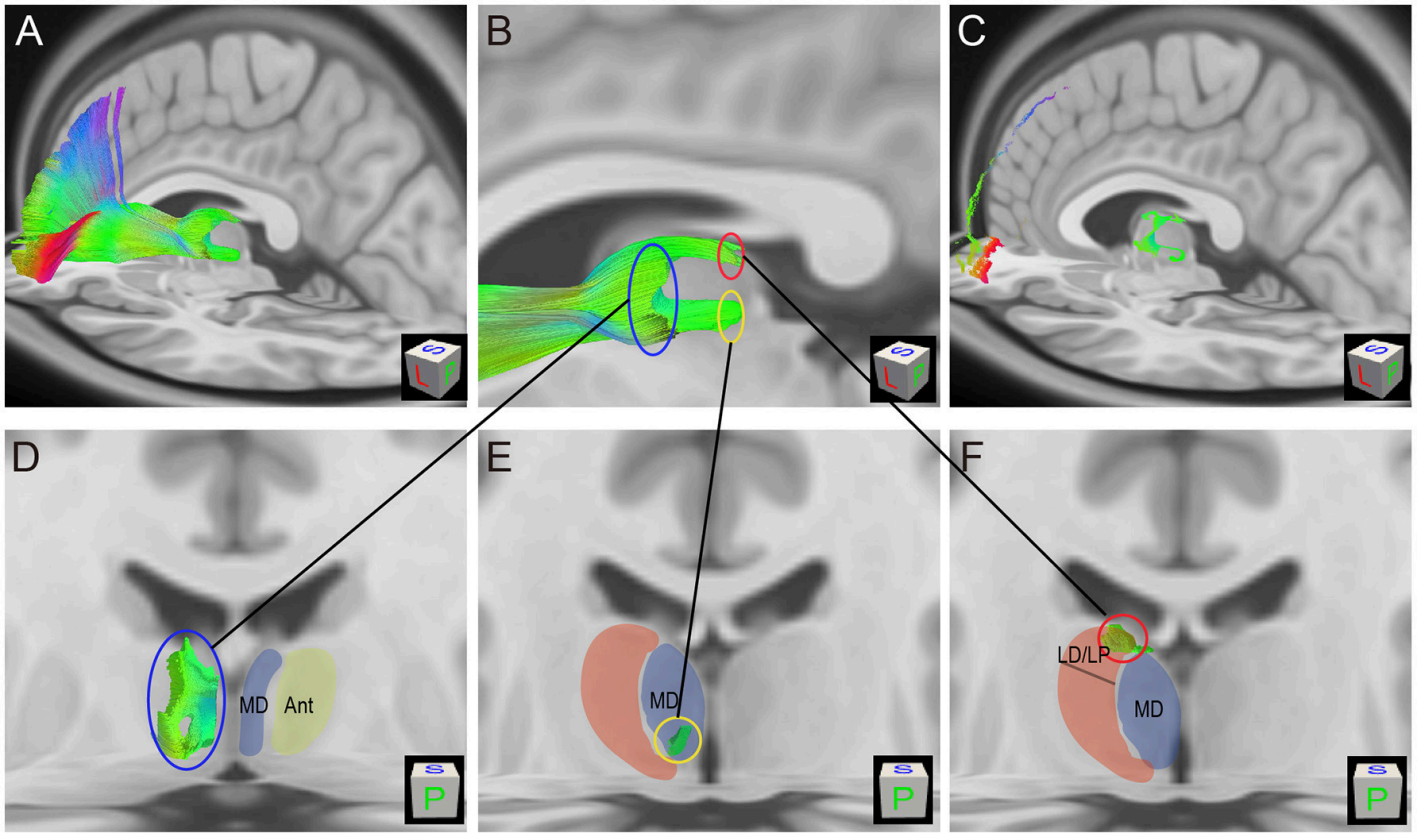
Thalamic-prefrontal peduncle (TPP) fiber origin/termination areas in the thalamus (HCP-842 template). (A) Lateral-posterior-superior view of the left TPP fibers. Fibers are overlaid on sagitial and axial T1 MRI slices. (B) The origin/termination areas in the thalamus are separated into three parts: dorsal (red circle), ventral (yellow circle), and anterior (blue circle). (C) The endpoints of TPP fibers are overlaid on T1 MRI slices. (D) The anterior origin/termination area of TPP fibers in the thalamus corresponds to the medial (MD) and anterior (Ant) nuclei of the thalamus. (E) The ventral origin/termination area corresponds to the ventral-posterior part of the MD. (F) The dorsal origin/termination area of TPP fibers in the thalamus corresponds to the lateral dorsal/lateral posterior nuclei (LD/LP).
